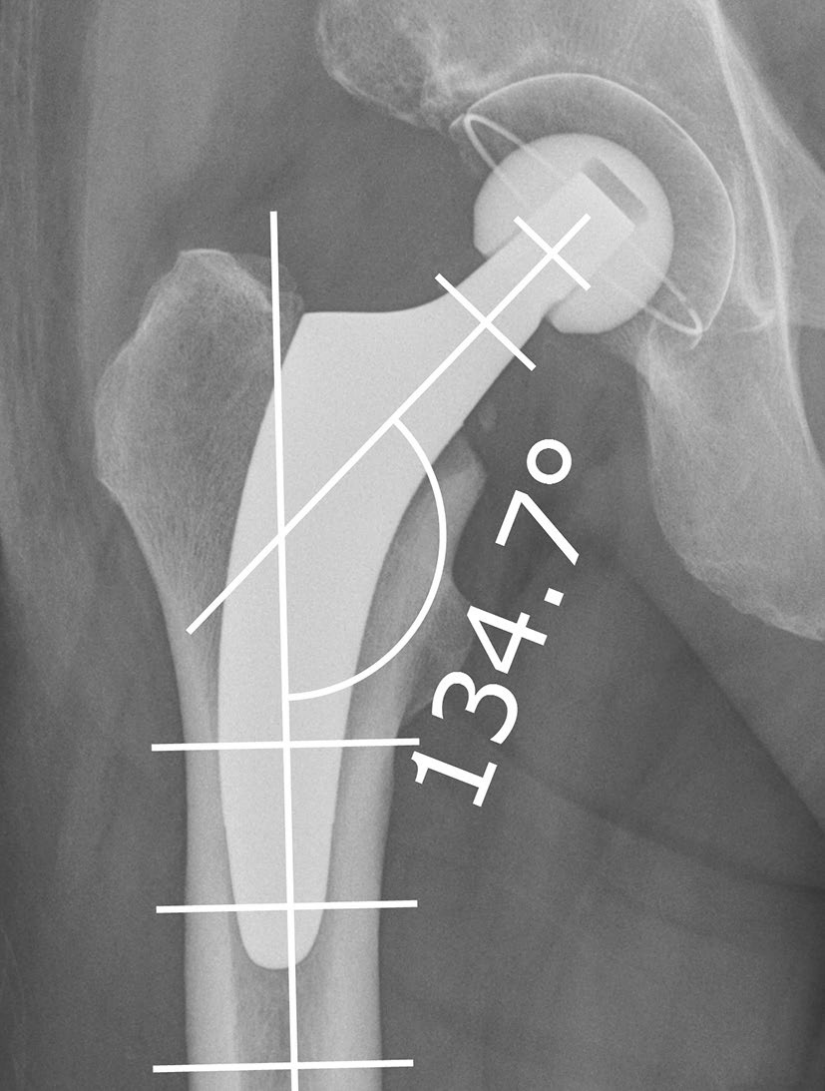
Measurement of CCD angle (between femoral axis and mid-neck-axis of the stem) on postoperative radiographs using the digital templating software MediCAD (Hectec, Landshut, Germany)
